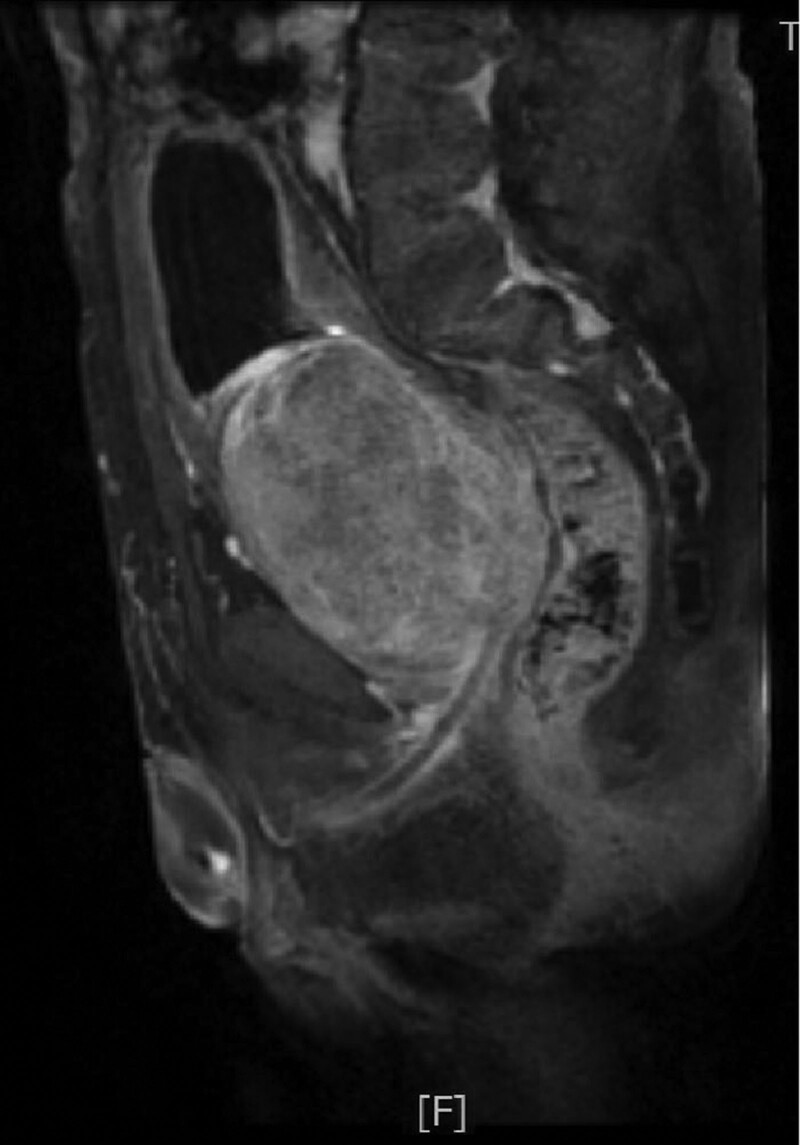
Sagittal plane of magnetic resonance image, huge prostate pressing on the rectum and bladder shifting upward.
